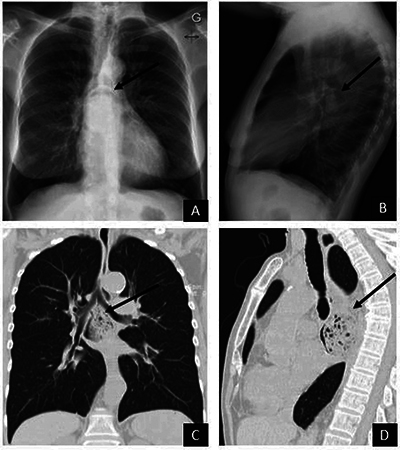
Chest radiography shows an AC in the middle mediastinum in anteroposterior (A) and lateral (B) views. A CT scan shows a foreign body mass within the thoracic oesophageal lumen (C) with an AC located anterior to the mass (D).
